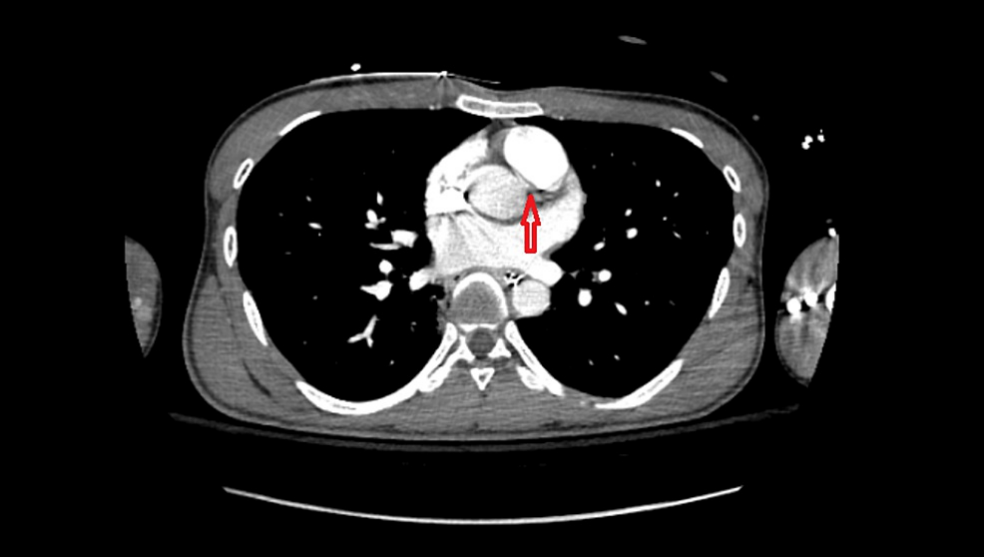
CT scan of the chest; note the vascular structure (arrow pointing) coursing between the aorta and the pulmonary trunk CT: computed tomography
